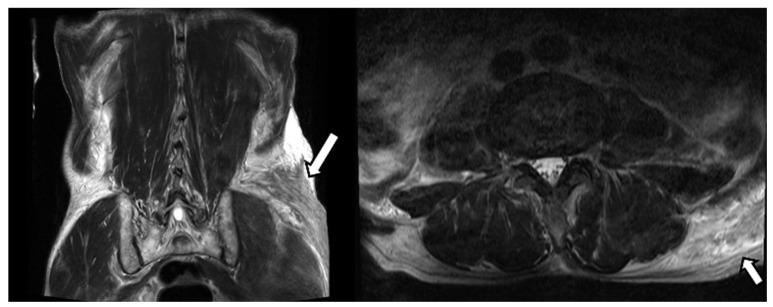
Magnetic resonance imaging (MRI) of the buttock. MRI revealed a well-defined mass in the left buttock. The mass showed a heterogeneously higher signal intensity than the skeletal muscle on T2-weighted images.
